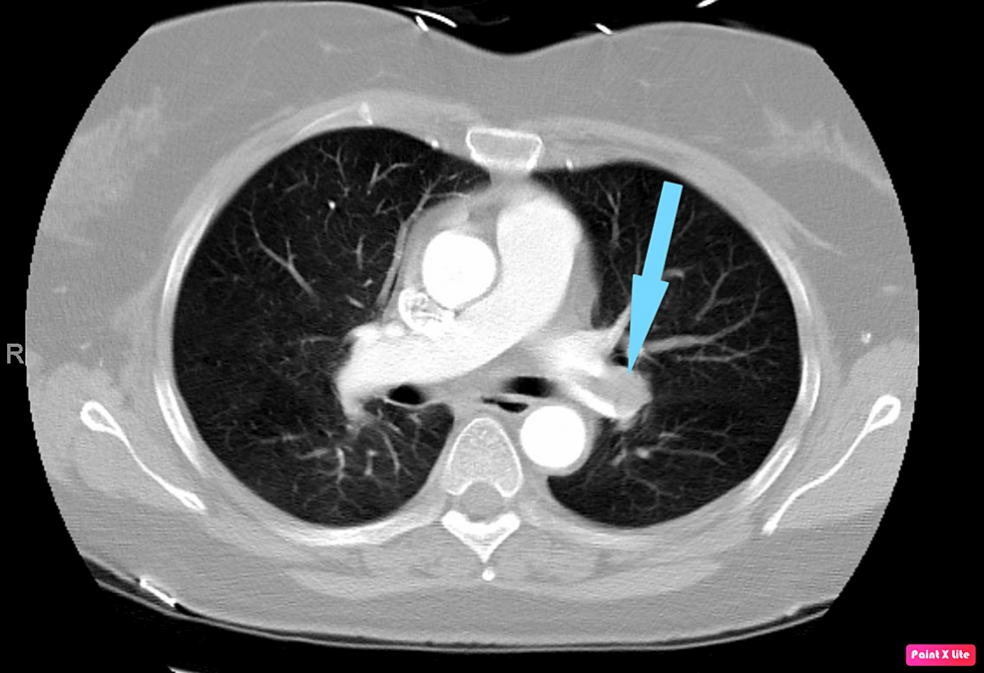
Thoracic CT showing left pulmonary artery embolus (blue arrow) CT: computed tomography
